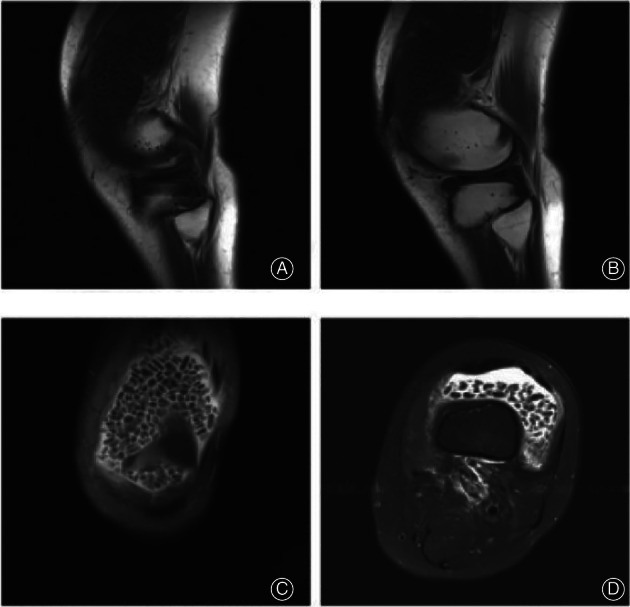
(A, B) Plain MRI scanning of the left knee joint. (A) MRT1WI sagittal knee joint. (B) MRT2WI sagittal knee joint. (C) MRT2 coronal knee joint. (D) MTR2 transverse knee joint.
